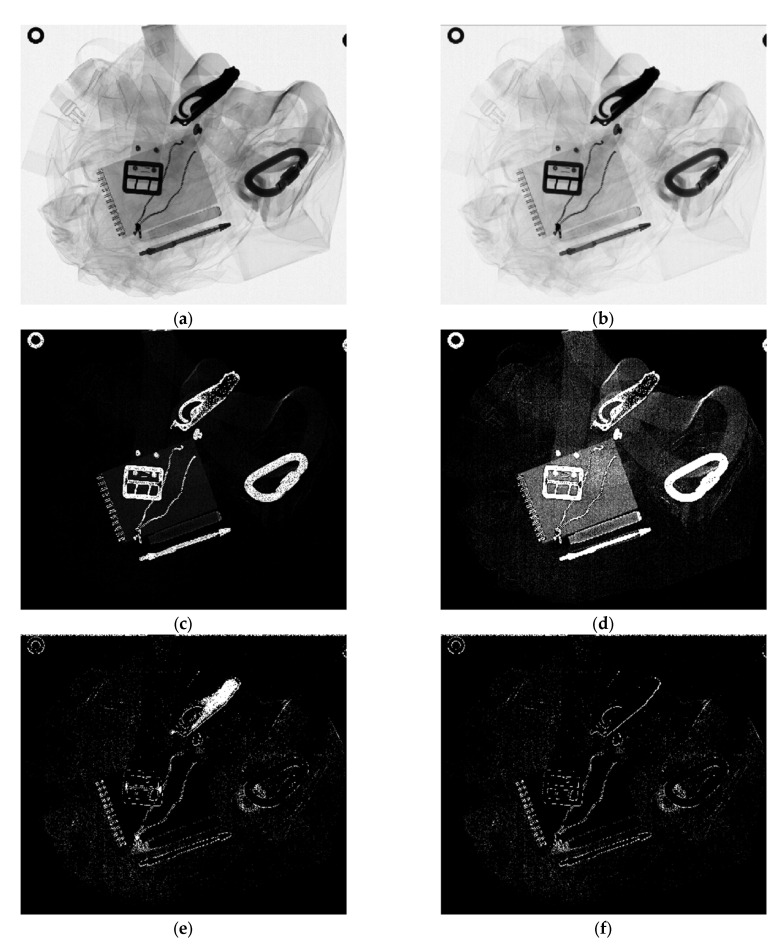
(a) Low-energy image of the paramedical trousers (adapted from [26], licensed under CC BY 4.0); (b) high-energy image; (c) areal density image with *Z*_eff_ = 26; (d) areal density image with *Z*_eff_ = 11.2; (e) areal density image with *Z*_eff_ = 6; (f) areal density image with *Z*_eff_ = 6 after removing areas of low X-ray transmission. As the second basis material, *Z*_eff_ = 7.1 was used in all cases.
